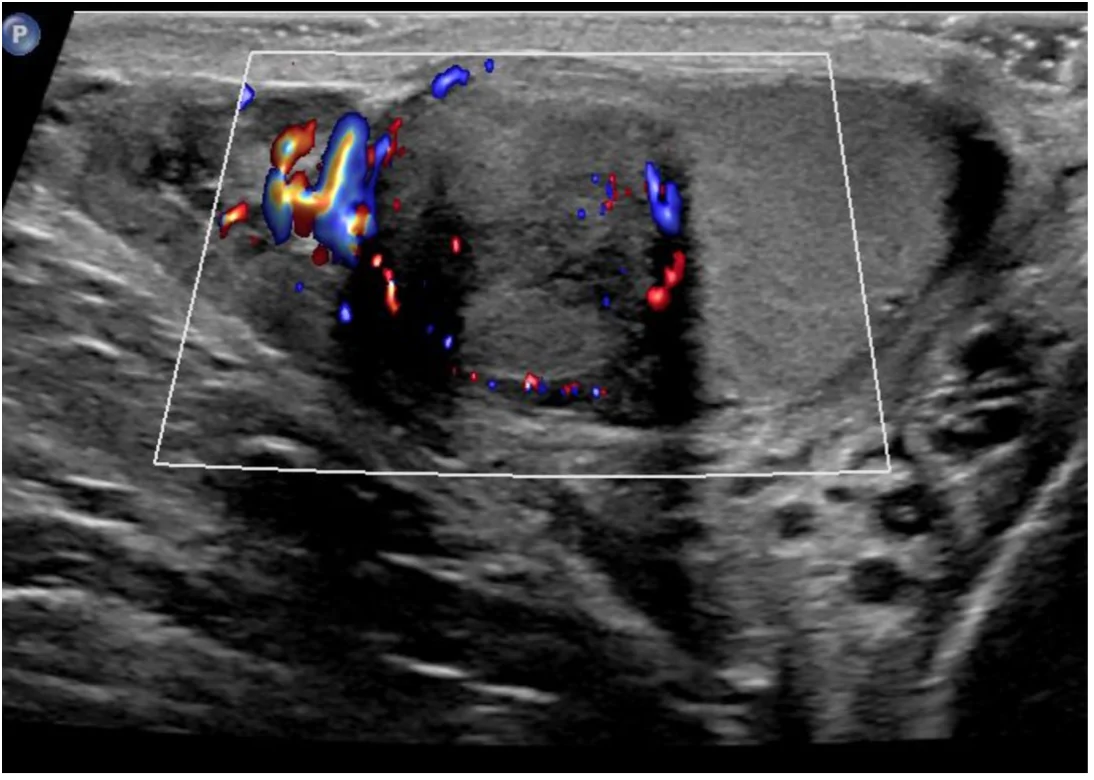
Color Doppler evaluation demonstrating internal vascularity, consistent with a vascular intratesticular lesion.
